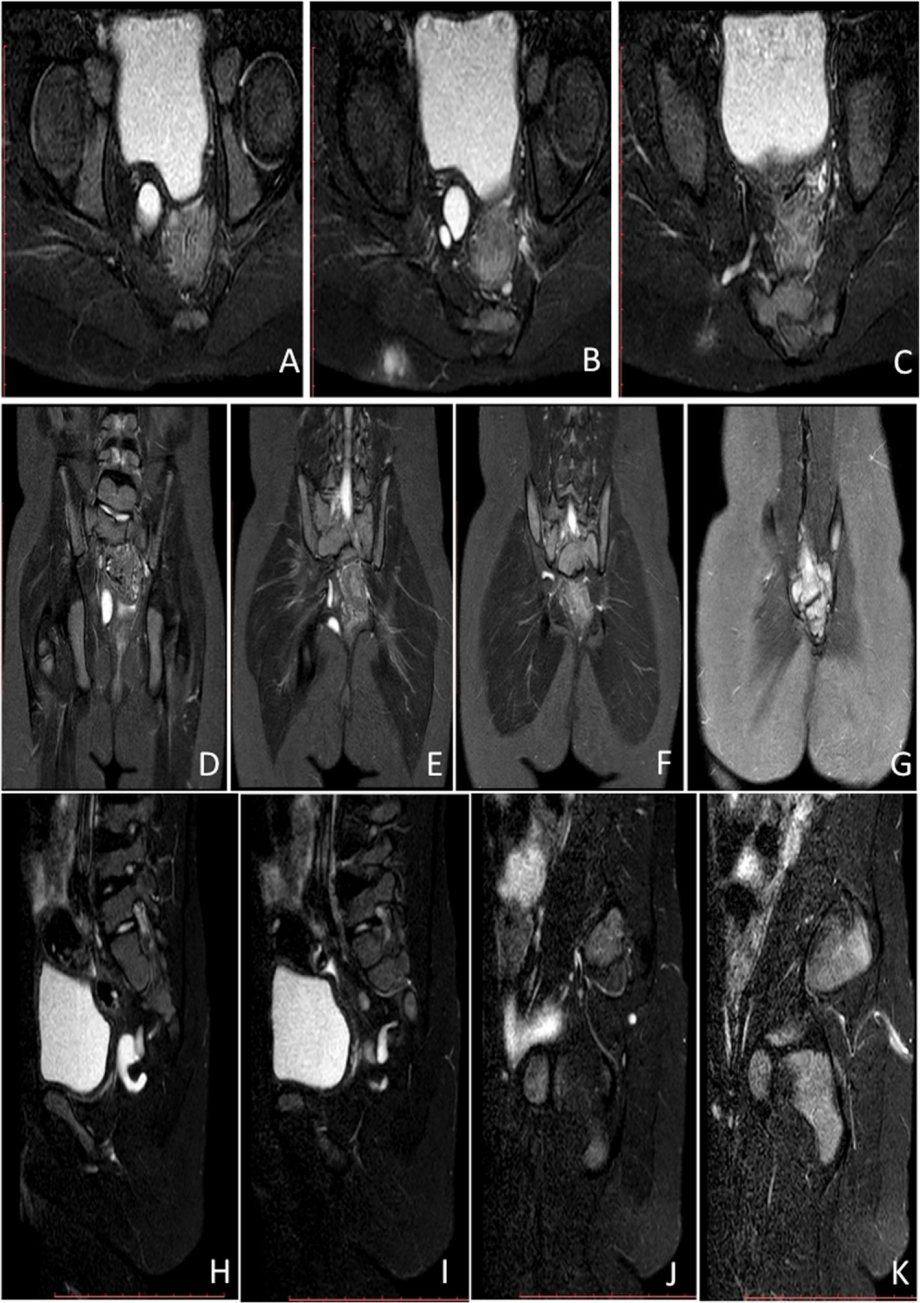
Axial (a-c), coronal (d-g), and sagittal (h-k) MRI on T2WI showed a sinus tract from the dimple to the retrorectal space, and the termination was a cyst
